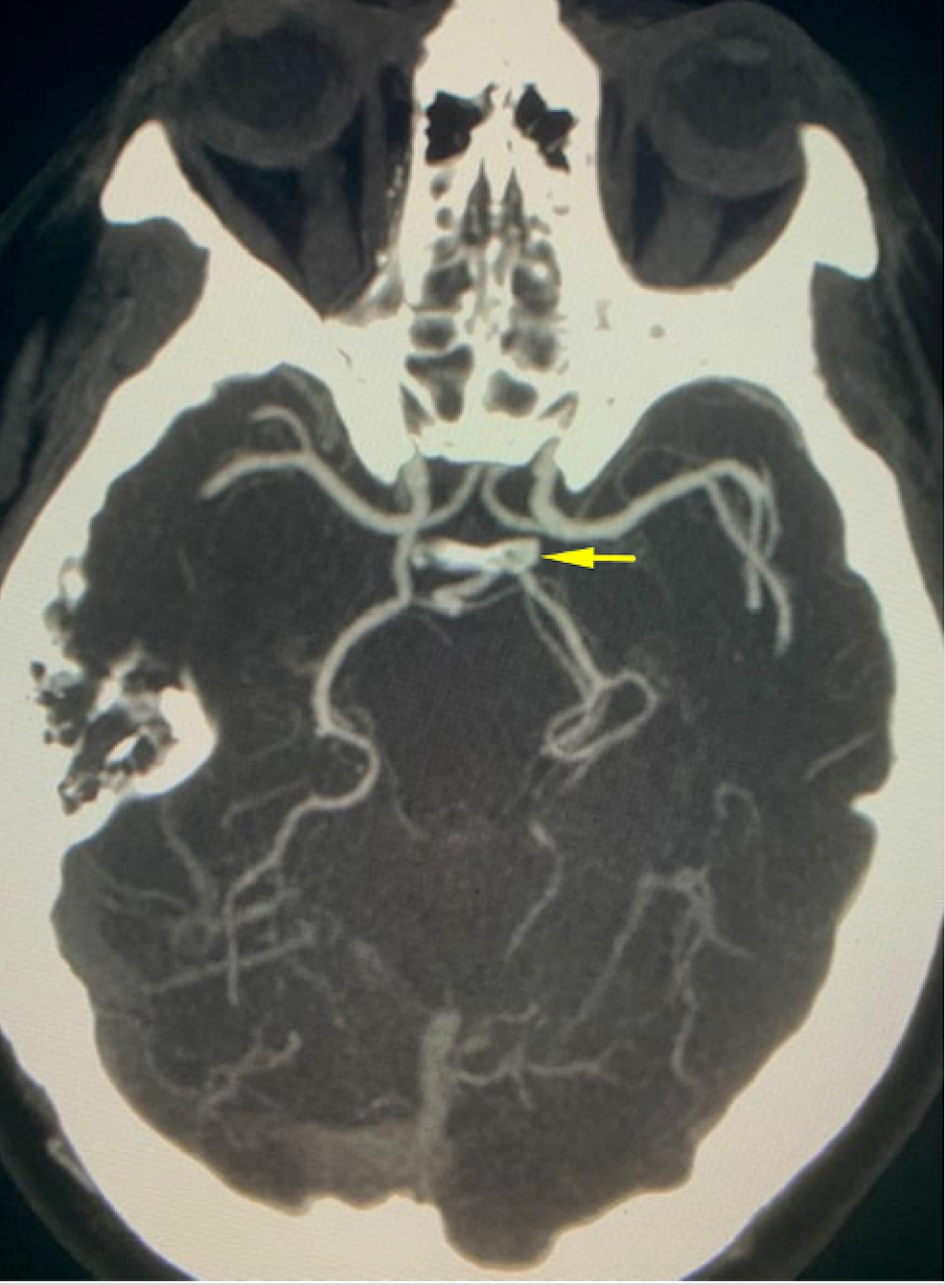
Axial CTA demonstrating fPCA CTA: computed tomography angiography; fPCA: fetal posterior cerebral artery
